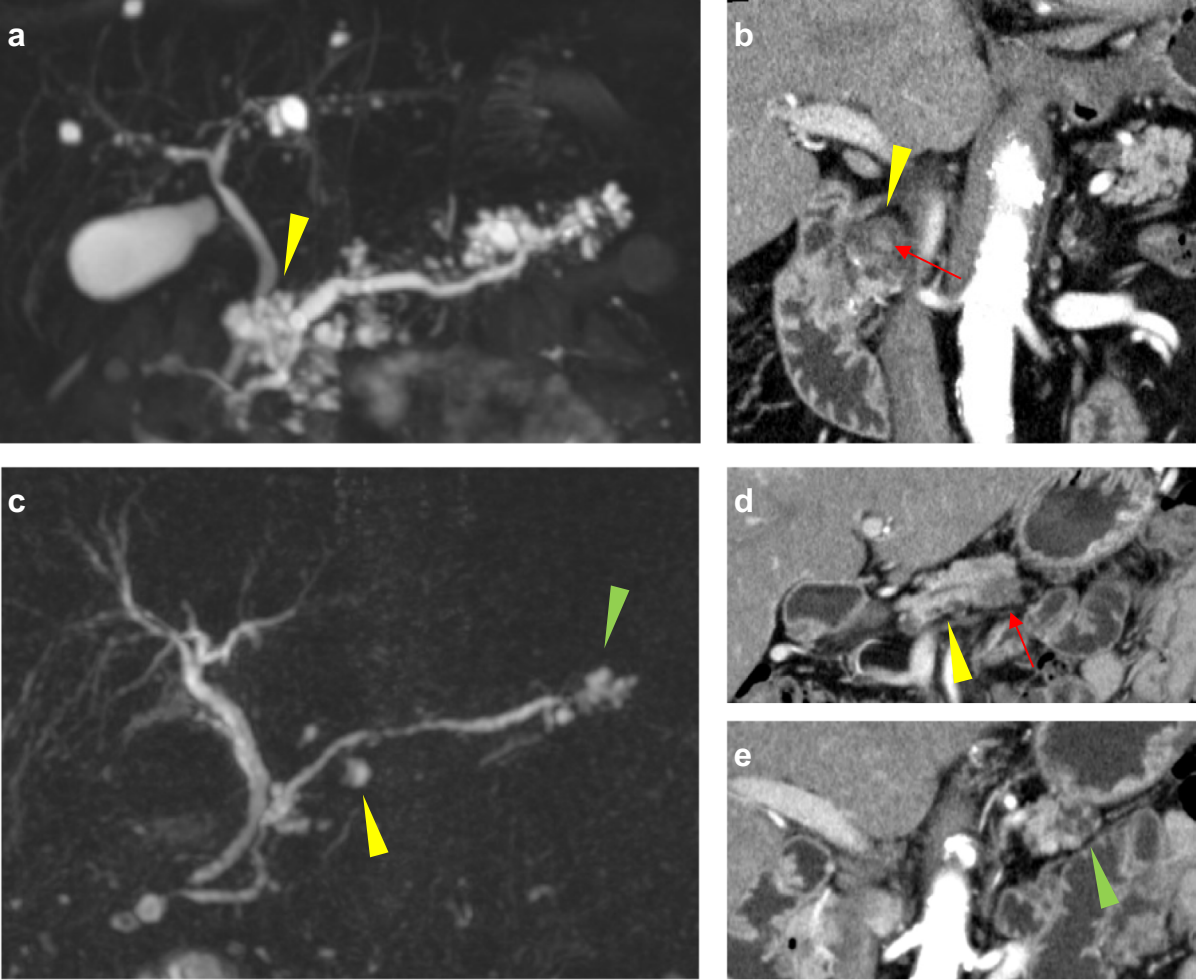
Patients diagnosed with pancreatic carcinomas during long-term surveillance of IPMNs. a and b Case with an IPMN-derived carcinoma. MRCP showed no mural nodule at the diagnosis of IPMNs at the whole pancreas (a). After follow-up of 7.8 years, contrast-enhanced CT showed a mural nodule in the pre-existing IPMN at the pancreatic head (b). The arrowhead in each figure indicates an IPMN, and the arrow indicates an enhancing mural nodule. c–e Case with a concomitant PDAC. Baseline MRCP showed several IPMN lesions at the body to tail of the pancreas without worrisome features or high-risk stigmata (c). After follow-up of 3.6 years, contrast-enhanced CT revealed a concomitant PDAC at the body of the pancreas (d and e). The arrowhead in each figure indicates an IPMN, and the arrow indicates a hypovascular mass suggestive of a concomitant PDAC. *CT* computed tomography, *IPMN* intraductal papillary mucinous neoplasm, *MRCP* magnetic resonance cholangiopancreatography, *PDAC* pancreatic ductal adenocarcinoma
